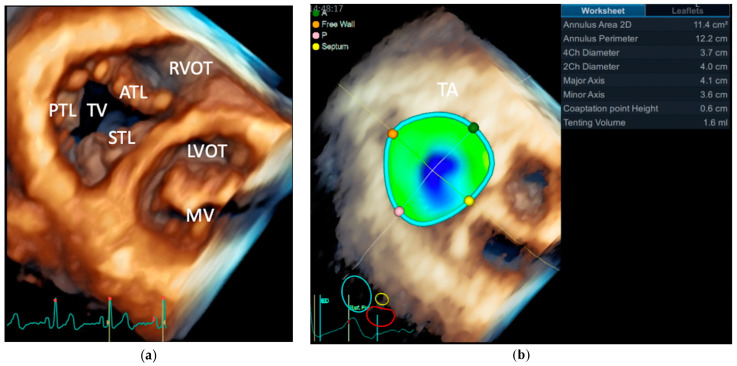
Transthoracic 3DE volume rendering of the tricuspid valve. (a) Anatomy of the tricuspid valve seen from the ventricular perspective and its relationships with adjacent structures; (b) Quantitative assessment of the tricuspid annulus. The colored dots on the annulus are used for anatomic orientation. Abbreviations: 2Ch, 2-chamber view; 2D, two-dimensional echocardiography; 3DE, three-dimensional echocardiography; 4Ch, 4-chamber view; A, anterior; ATL, anterior tricuspid leaflet; LVOT, left ventricular outflow tract; MV, mitral valve; P, posterior; PTL, posterior tricuspid leaflet; RVOT, right ventricular outflow tract; STL, septal tricuspid leaflet; TA, tricuspid annulus; TV, tricuspid valve.
